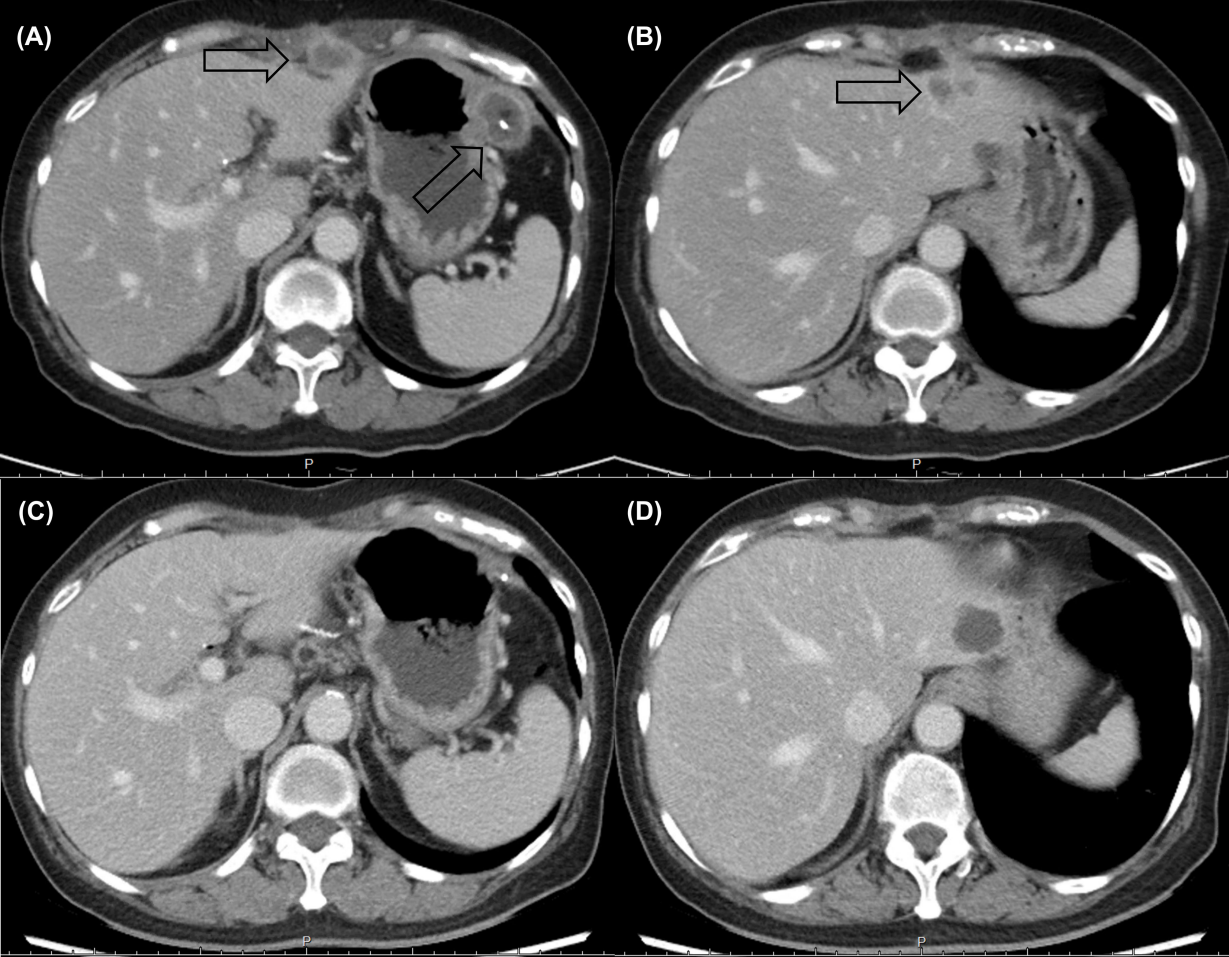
Computed tomography revealed multiple rim-enhancing (A) peritoneal metastases and (B) liver metastases before low-dose nivolumab. A durable complete response (C, D) was maintained after more than 1 year of nivolumab-based treatment.
